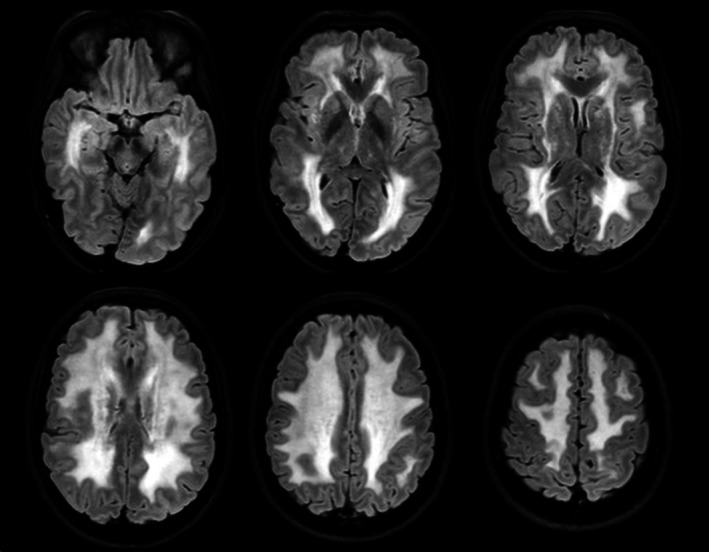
Neuroradiological findings. Axial T2 fluid‐attenuated inversion recovery imaging (FLAIR) shows diffuse, confluent and symmetric severe supratentorial white matter hyperintensities with sparing of U fibers and corpus callosum.
